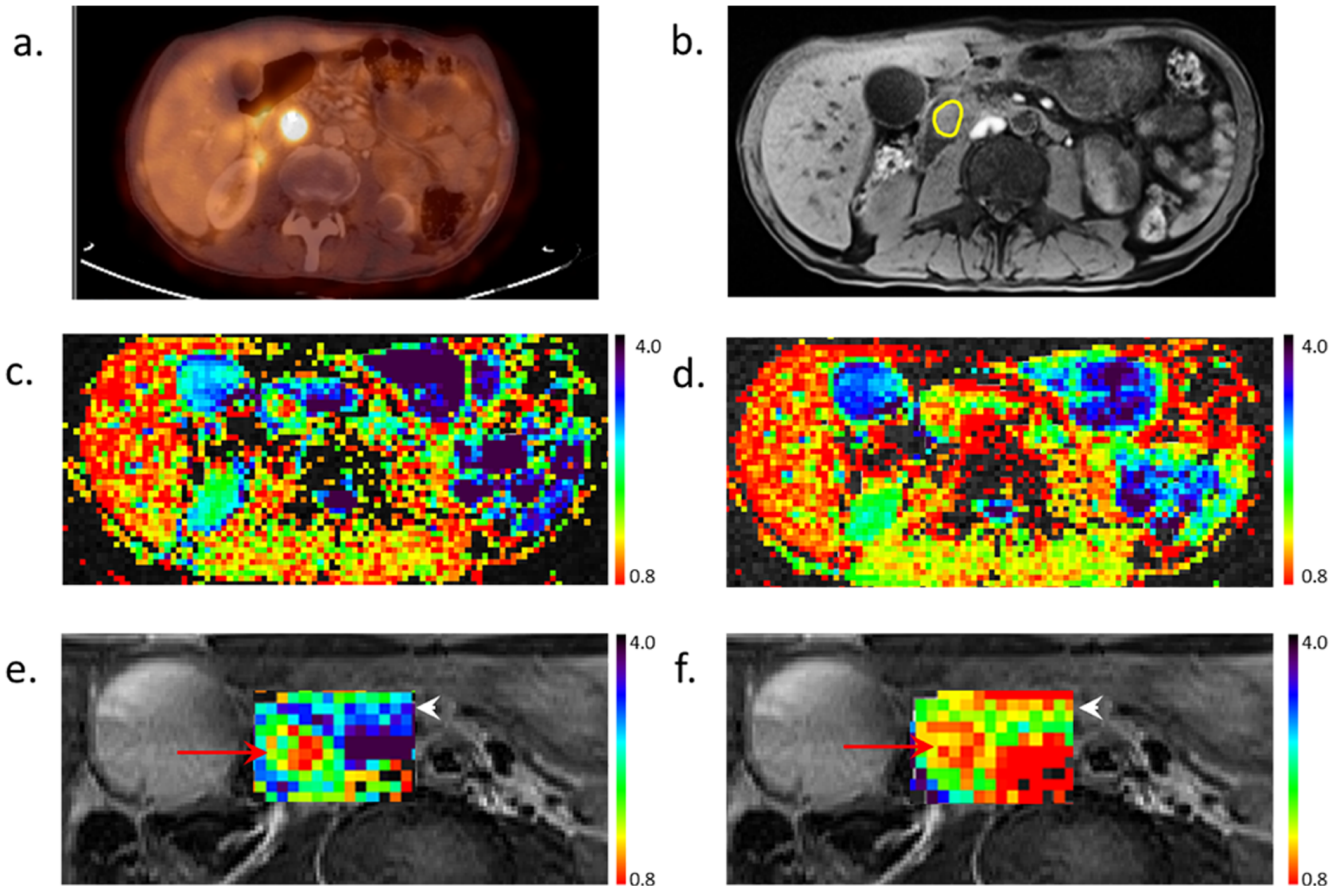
PET-CT, DCE-MRI and ADC maps using b-values 0,500 s/mm^2^ and 100, 500 s/mm^2^ in the region of PDAC of a male patient (age 66). (a) PET-CT exhibiting increased uptake of ^18^F labeled deoxy-glucose (FDG) in the region of pancreatic head (b) T1-weighted, pre-contrast image revealing PDAC location, marked by yellow circle. (c and d) ADC maps of the entire abdominal slice using b-values of 0, 500 s/mm^2^ and 100, 500 s/mm^2^, respectively. (d and e) Zoomed (x2) ADC maps of the regions in c and d that include PDAC and its surrounding, using b-values of 0, 500 s/mm^2^ and 100, 500 s/mm^2^, respectively, overlaid on the corresponding T2-weighted image. *Notice* that the tumor in e (red arrow) is clearly visible on the 0, 500 s/mm^2^ ADC map showing a high contrast with the surrounding normal tissue (white arrow head). However, in f, due to the reduction in ADC of the normal at 100, 500 s/mm^2^ (from 2.22±0.64 to 1.49±0.35 mm^2^/s) and the similarity in the ADC of the tumor (from 1.38±0.37 to 1.24±0.28 mm^2^/s) the contrast between the tumor and the surrounding pancreatic tissue is diminished.
